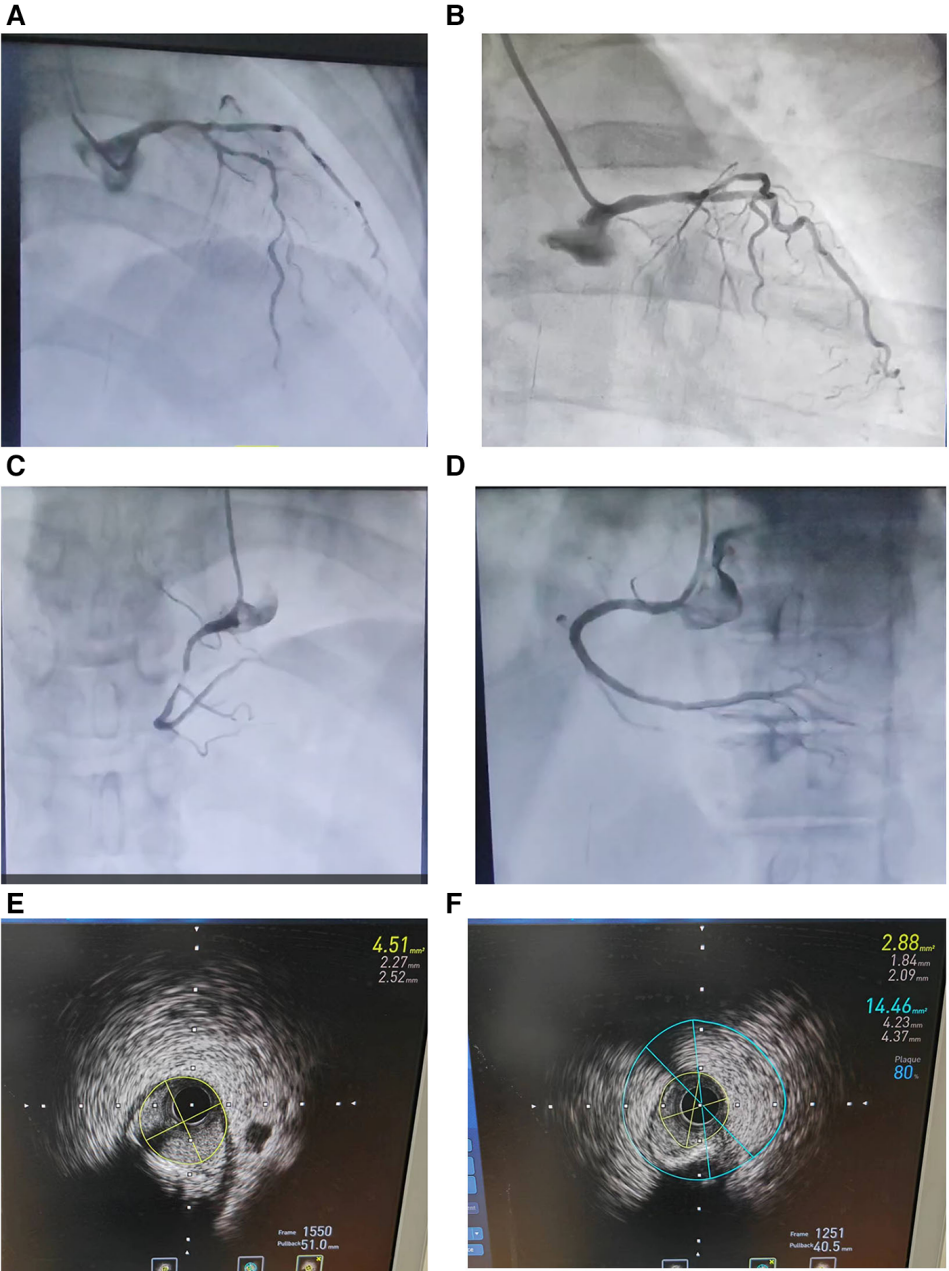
Coronary angiography (A–D) and intravascular ultrasound examination (E and F) in an elderly patient with Sheehan's syndrome. (A) The stenosis degree is 40%, 80%, and 60%, respectively, at the end of the left main trunk, the proximal and middle segments of the left anterior descending branch. (B) The stenosis degree is 98% at the proximal segments of the left circumflex branch. (C) The stenosis degree is almost completely occluded at the middle segment of the right coronary artery. (D) Two stents are inserted in the middle segment of the RCA. (E) The minimum lumen area at the distal stenosis of the left main trunk is 4.51 mm^2^. (F) The plaque load at the most severe stenosis of the proximal left anterior descending branch is 80%, and the minimum lumen area is 2.88 mm^2^.
